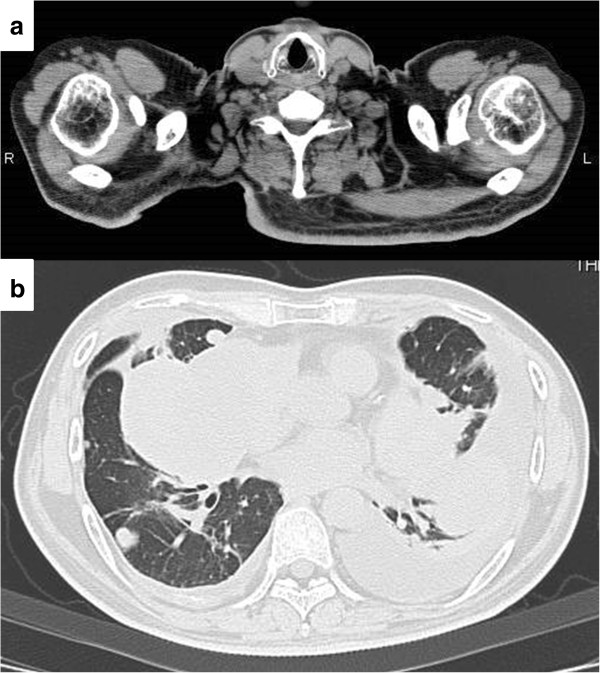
CT imaging 14 months after resection of the muscle metastasis. (a) No local recurrence was observed. (b) The pulmonary metastases began to grow rapidly.
